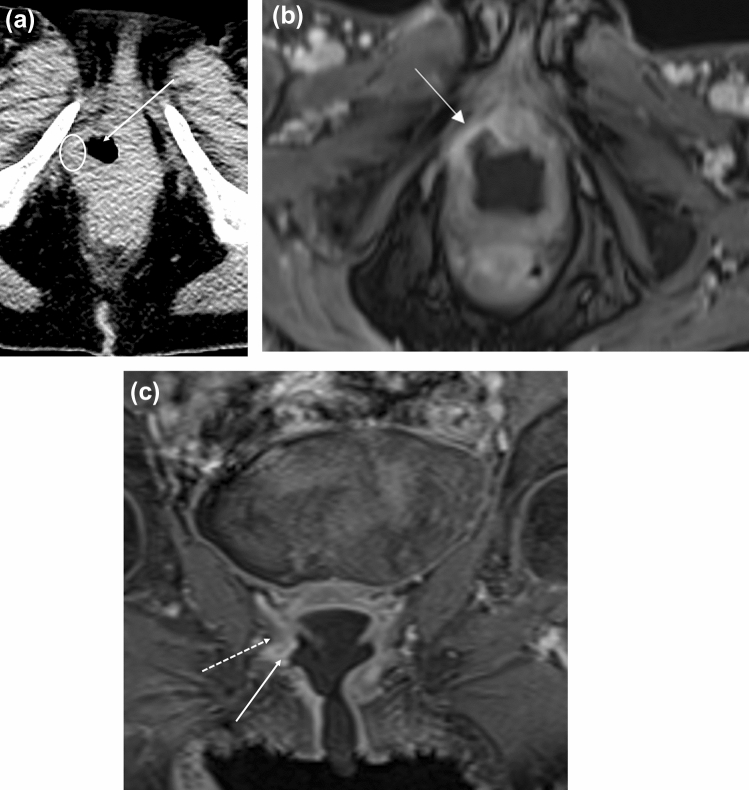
58-year-old woman with FIGO stage 3 squamous cell carcinoma of the vagina who was treated with chemoradiation. a Axial CT before treatment shows an ulcerated mass in the right vaginal wall (arrow). There is a subtle area of hypodensity representing the non-ulcerated portion of the mass (circle). b Axial T1-weighted contrast-enhanced MR image after treatment shows enhancing tissue (arrow) compatible with residual tumor. c Coronal T1-weighted contrast-enhanced MR image shows that the lesion (solid arrow) is predominantly infralevator in location but extends superiorly to involve the levator ani muscle (dotted arrow)
